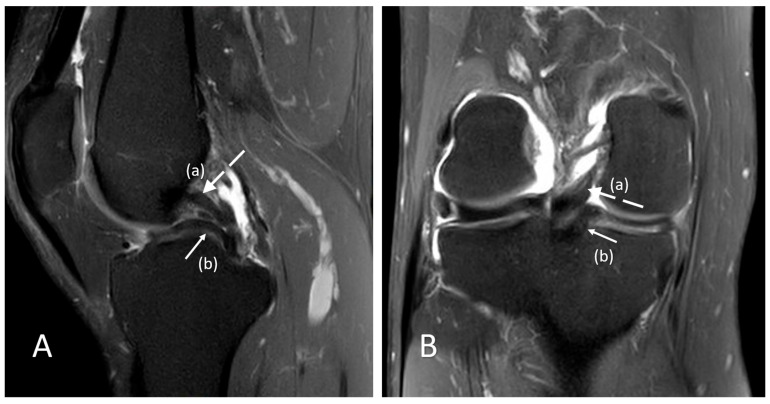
A 34-year-old man with a body mass index (BMI) = 24.6 kg/m^2^ sustained a rotational in-jury of the left knee during athletic activity (basketball practice) and subsequently developed persistent medial joint-line pain. Three months later, he continued to report mechanical symptoms with a limitation of knee extension of approximately 7°. Clinical examination demonstrated medial joint-line tenderness, while both the McMurray and Thessaly tests were positive, raising suspicion of a meniscal injury. MRI was performed on a 1.5 T scanner using a sagittal (A) and coronal (B) proton density fat-suppressed (PD-FS) sequence of the knee. Sagittal PD-FS images (Panel (A)) demonstrate a low-signal, curvilinear structure within the intercondylar notch running parallel and immediately anterior to the posterior cruciate ligament (PCL) [white dashed arrow (a)], producing the classic double PCL sign. This structure corresponds to a displaced bucket-handle fragment of the medial meniscus [white arrow (b)]. Coronal PD-FS images [Panel (B): white dashed arrow (a): PCL, (b): displaced medial meniscus fragment] confirm displacement of the torn medial meniscal fragment into the intercondylar notch [white arrow (b)] with associated loss of the normal medial meniscal body configuration. These findings are characteristic of a displaced bucket-handle tear of the medial meniscus, explaining the patient’s persistent mechanical symptoms, and arthroscopic surgical treatment was subsequently proposed. Although the double PCL sign is a well-recognized MRI finding, its recognition remains clinically important, as it represents a highly specific, though not pathognomonic, indicator of a displaced bucket-handle meniscal tear requiring timely surgical management. The sign corresponds to a displaced meniscal fragment within the intercondylar notch lying anterior and parallel to the intact PCL, creating the characteristic appearance [1,2,3,4,5]. Potential diagnostic pitfalls include the meniscofemoral ligaments (ligaments of Humphrey and Wrisberg), discoid medial meniscus, mucoid degeneration of the PCL (“tram-track” appearance), and other intercondylar structures; therefore, it should be interpreted in the appropriate clinical and imaging context [6,7,8,9].
